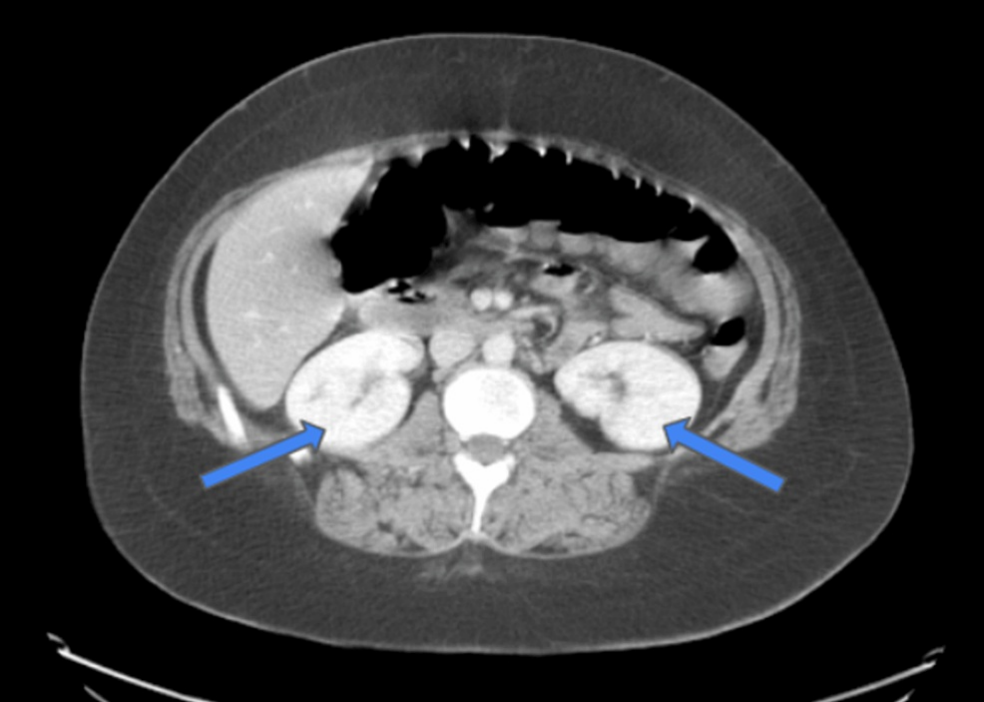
CT of the abdomen/pelvis with IV contrast at presentation showed bilateral multifocal scarring of the kidneys with superimposed pyelonephritis (blue arrows)
